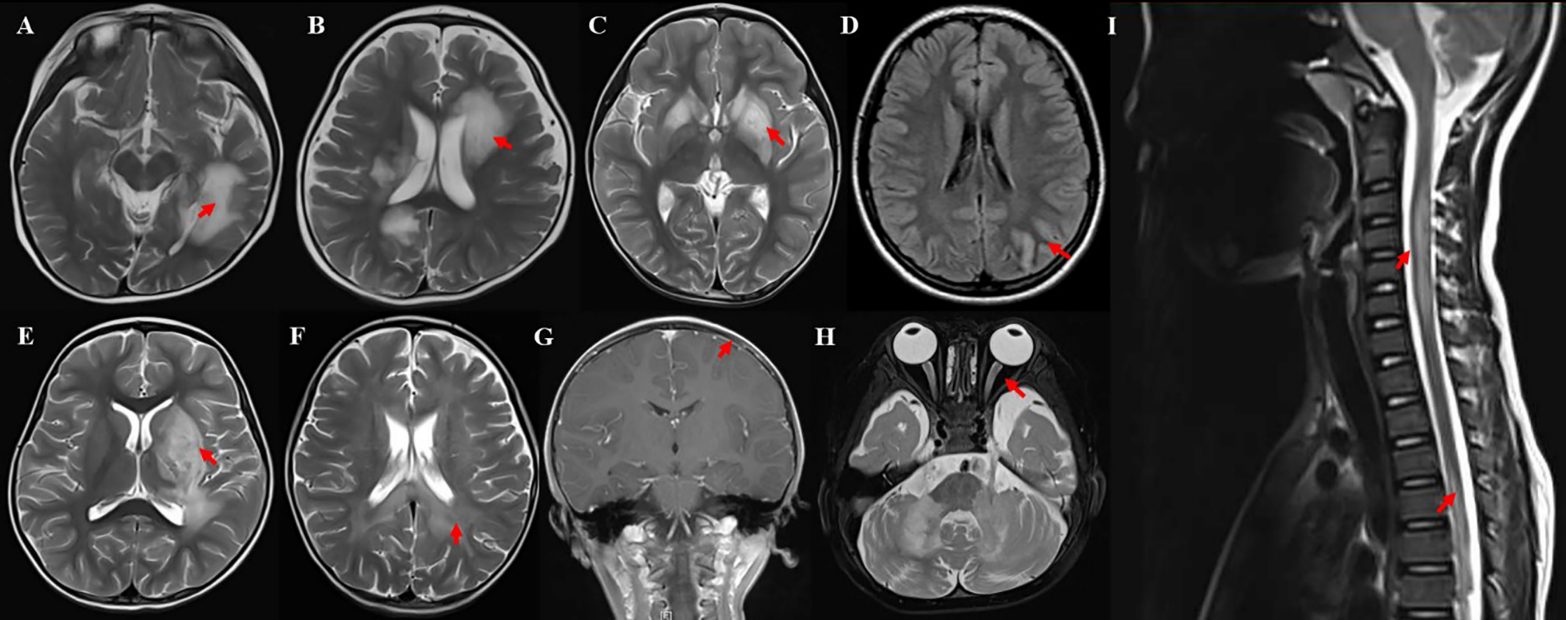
Example of MRI of MOGAD patients. (A) Large, poorly demarcated in subcortical white matter; (B) MS-like lesion around in periventricular; (C) Bilateral basal ganglia obvious edema, similar to EBV encephalitis; (D) Left occipital and temporal cortex edema with T2WI-hyperintense lesions along the gyrus; (E) Tumefactive lesion in left basal ganglia; (F) Symmetric white matter lesions around the posterior horn of lateral ventricle (leukodystrophy-like); (G) Local leptomeningeal gadolinium enhancement in T1WI; (H) bilateral optic edema; (I) longitudinally extensive transverse myelitis in the cervical and thoracic spinal cord.
